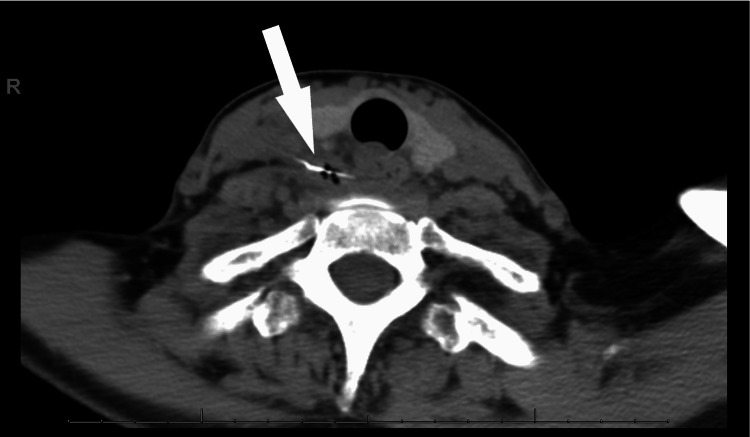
Axial computed tomography image displaying the wire bristle with evidence of esophageal perforation
